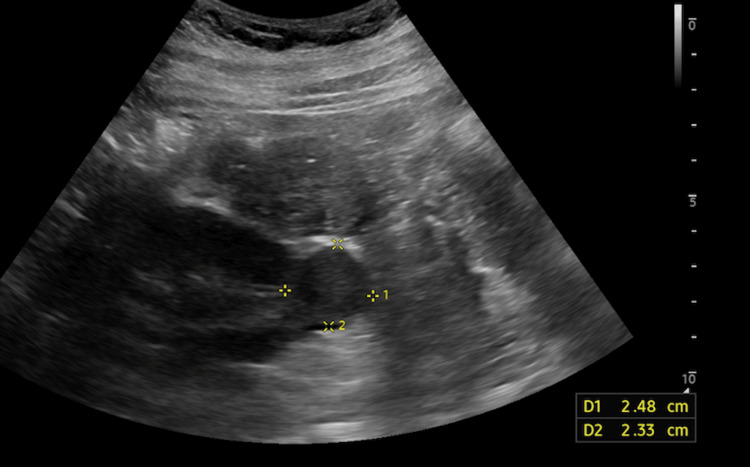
Transabdominal grayscale and duplex sonography of the retroperitoneum
